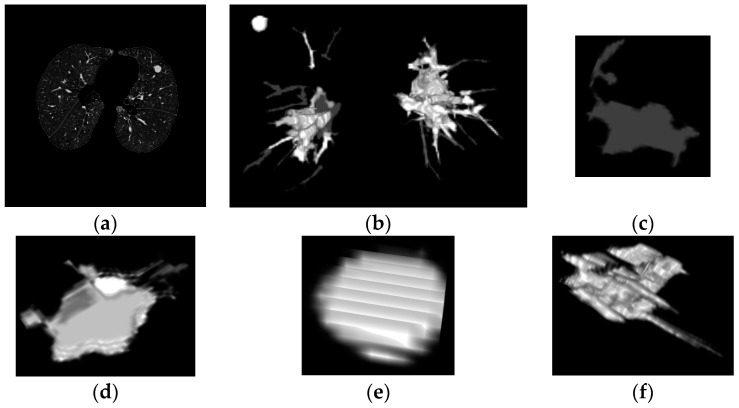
Lung nodule detection with false positives (a) CT image showing all the structures; (b) Lung 3D reconstruction; (c–f) all possible candidates before classification; (c,d) Non-nodules; (e) lung nodules determine after classification; (f) False positive presented in the detection.
